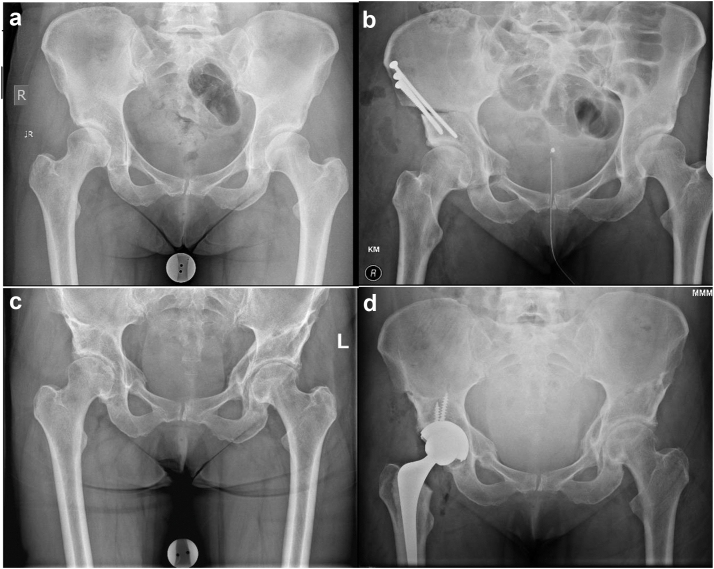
Plain pelvis and both hips AP x-rays. (a) Preoperative x-rays at age of 46 years, (b) Immediate postoperative right PAO, (c) 8 years post right PAO, (d) Right THA postoperative x-rays at 9 years post-PAO. AP, anterior-posterior.
